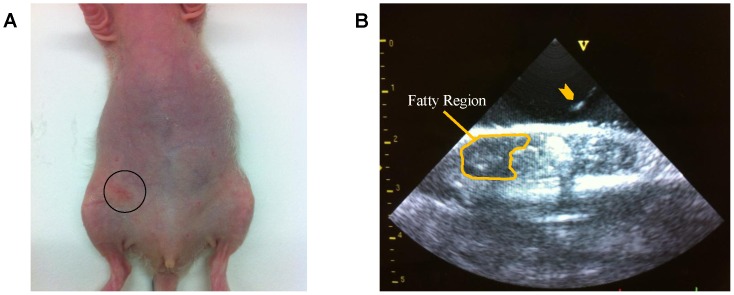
Cell inoculation under ultrasound image guidance. (A) Cancer cells were injected through the skin (circle) and into the lower mammary fat pad under ultrasound image guidance. (B) Ultrasound image showing needle tip (arrowhead) and mammary fat pad.
